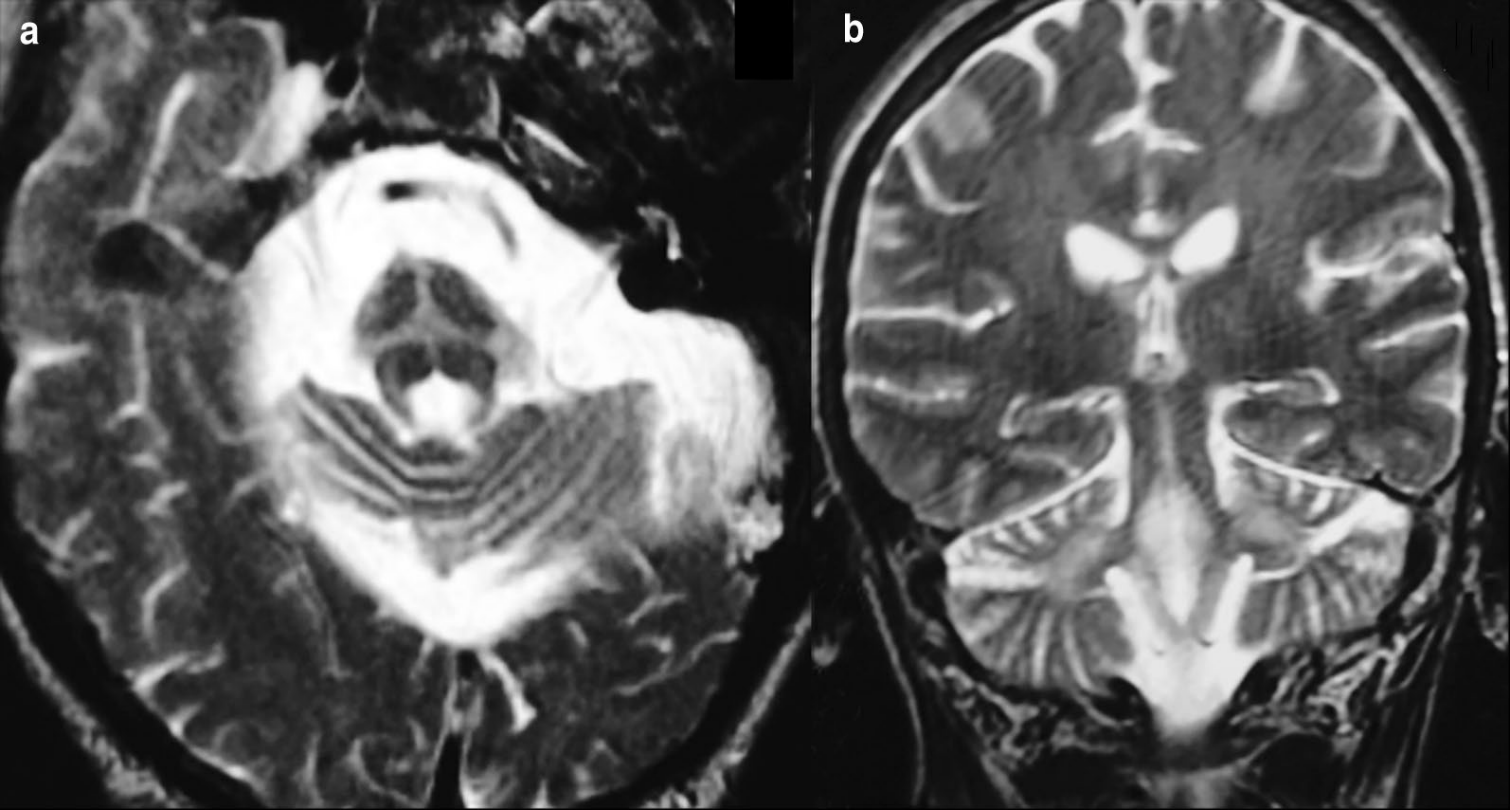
Axial T2-weighted image demonstrates cruciform hyperintense signal in the pons, the so-called hot cross bun sign of MSA-C (a). Coronal T2-weighted image at the level of the brainstem demonstrates hyperintense signal in the bilateral brachis pontis, another common finding in the disease (b)
